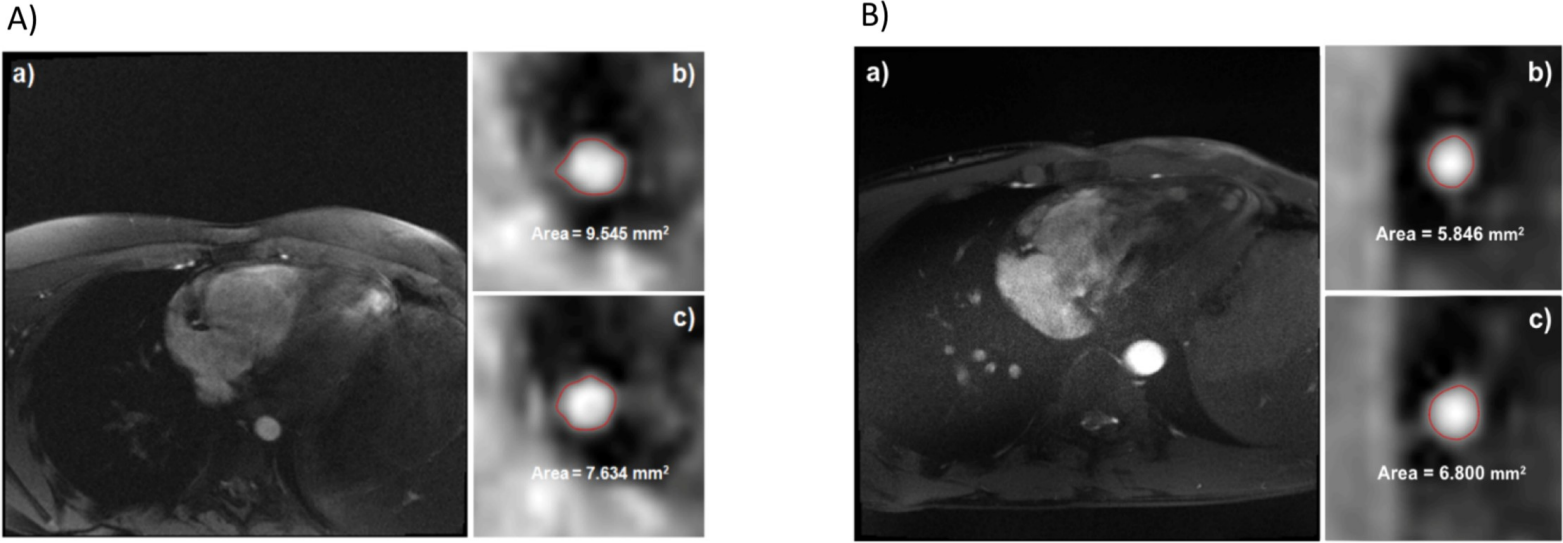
Representative MR images. Representative MR anatomical and flow velocity images during measurement of coronary vasomotor response of the right coronary artery (RCA) to handgrip exercise. Representative images obtained from a child with A) T1DM (left panel) and B) a healthy control (right panel). (a) double oblique scout scan obtained in parallel to the RCA. (b) cross-sectional images of the RCA acquired at rest (baseline). (c) cross-sectional images of the RCA during isometric handgrip stress. The vessel lumen area is represented by the red line.
